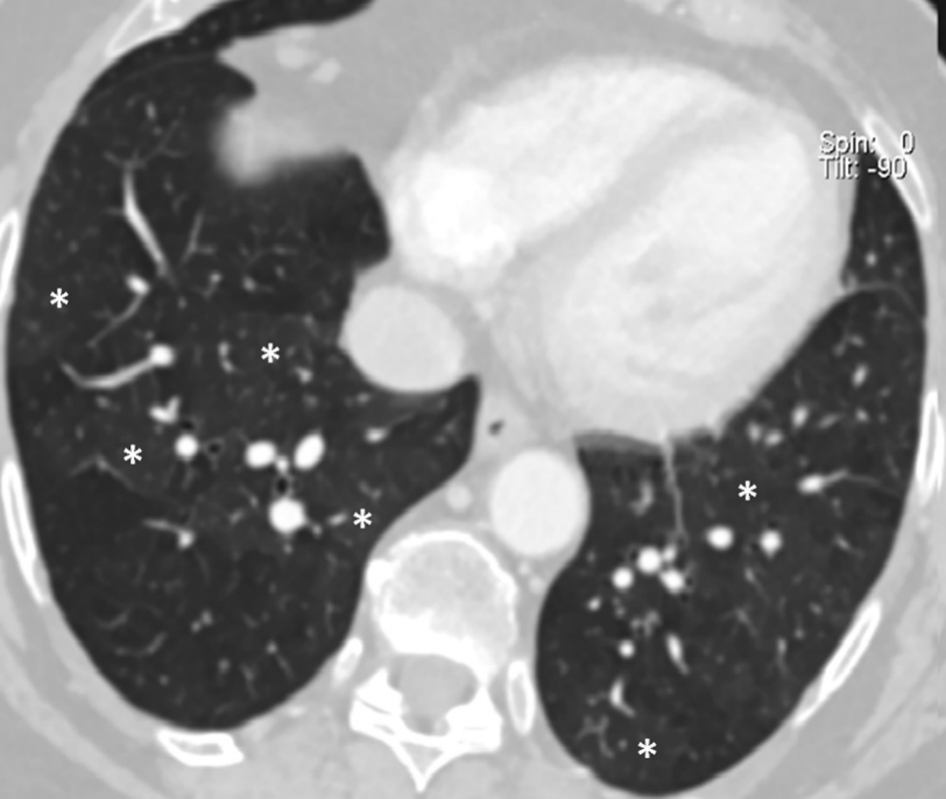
Thoracic CT-scan in inspiration: 1 year after the onset lung micronodules
